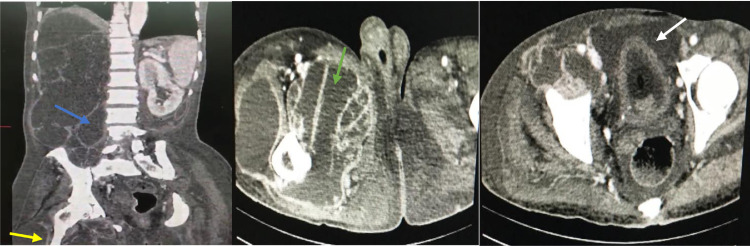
Abdominal and pelvic enhanced-contrast CT: sagittal reconstruction (A) and in transverse sections (B and C) showing collections with the same density as that described above in the right iliac psoas (blue arrows), the right gluteal (yellow arrow) and adductors (green arrow) muscles with low pelvic peritoneal effusion (white arrow).
